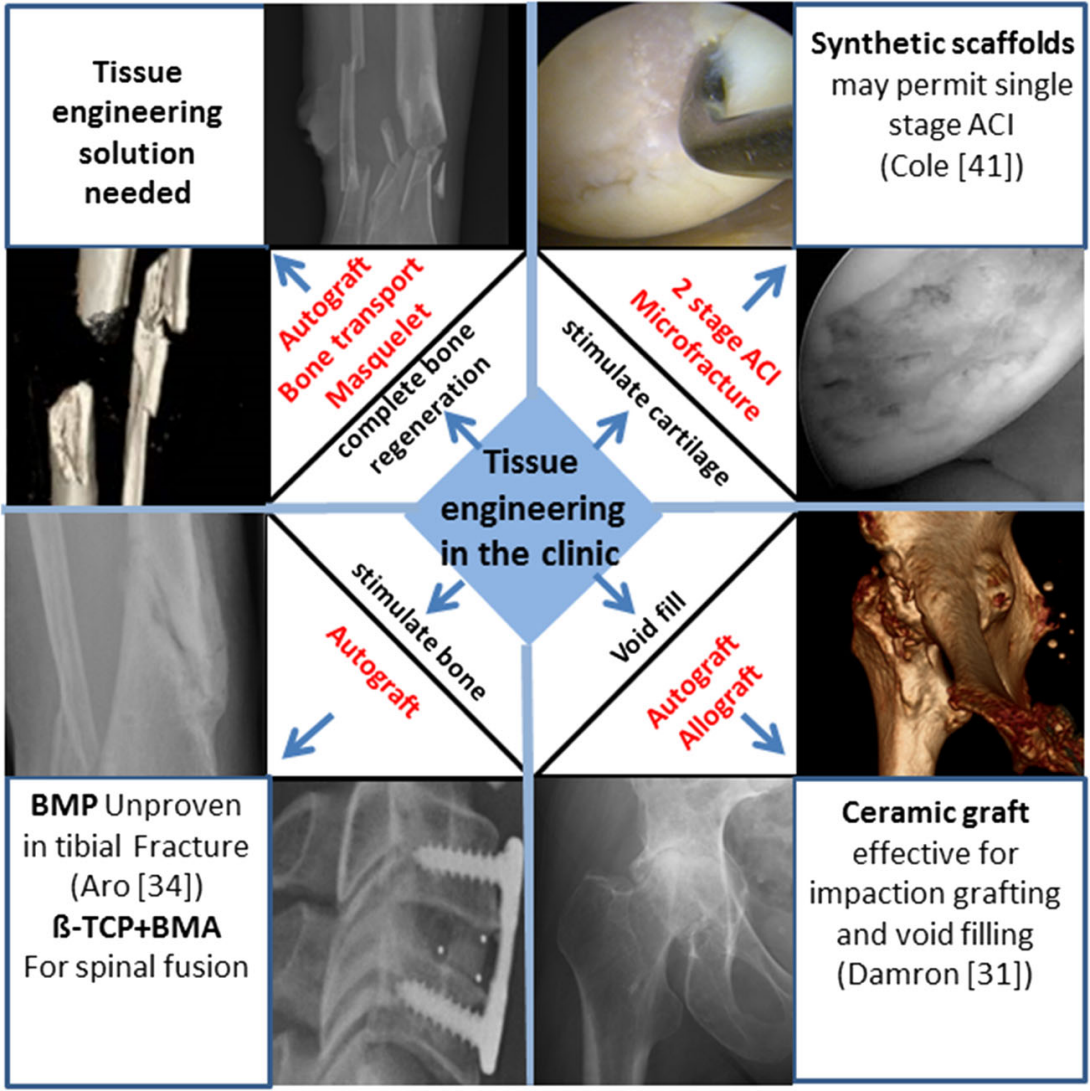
Skeletal tissue engineering in the clinic. Clinical skeletal repair requirements: (i) cartilage regeneration, (ii) bone void filling, (iii) stimulation of fracture healing or arthrodesis and (iv) reconstruction of segmental bone loss are represented in different quadrants. (i) In the *top right quadrant* are arthroscopic images of an osteochondral lesion (*upper*) and chondral lesion post microfracture (*lower*). (ii) In the *lower right quadrant* is a 3D reconstruction (*upper*) and radiograph (*lower*) of a patient with severe osteoarthritis and protrusio acetabuli. (iii) In the *lower left quadrant* show radiographs demonstrating fracture non-union and spinal arthrodesis. (iv) In the *top left quadrant* demonstrates a comminuted tibial fracture (*upper*) and segmental bone defect (*lower*). Current treatment strategies are detailed (in *red*) in corresponding *triangles*
